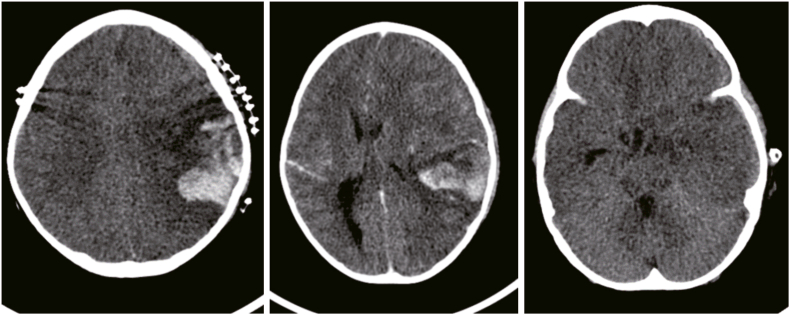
Axial contrasted CT of the brain showing diffuse brain injury with left temporal-parietal intracranial haemorrhage and associated mass effect.
